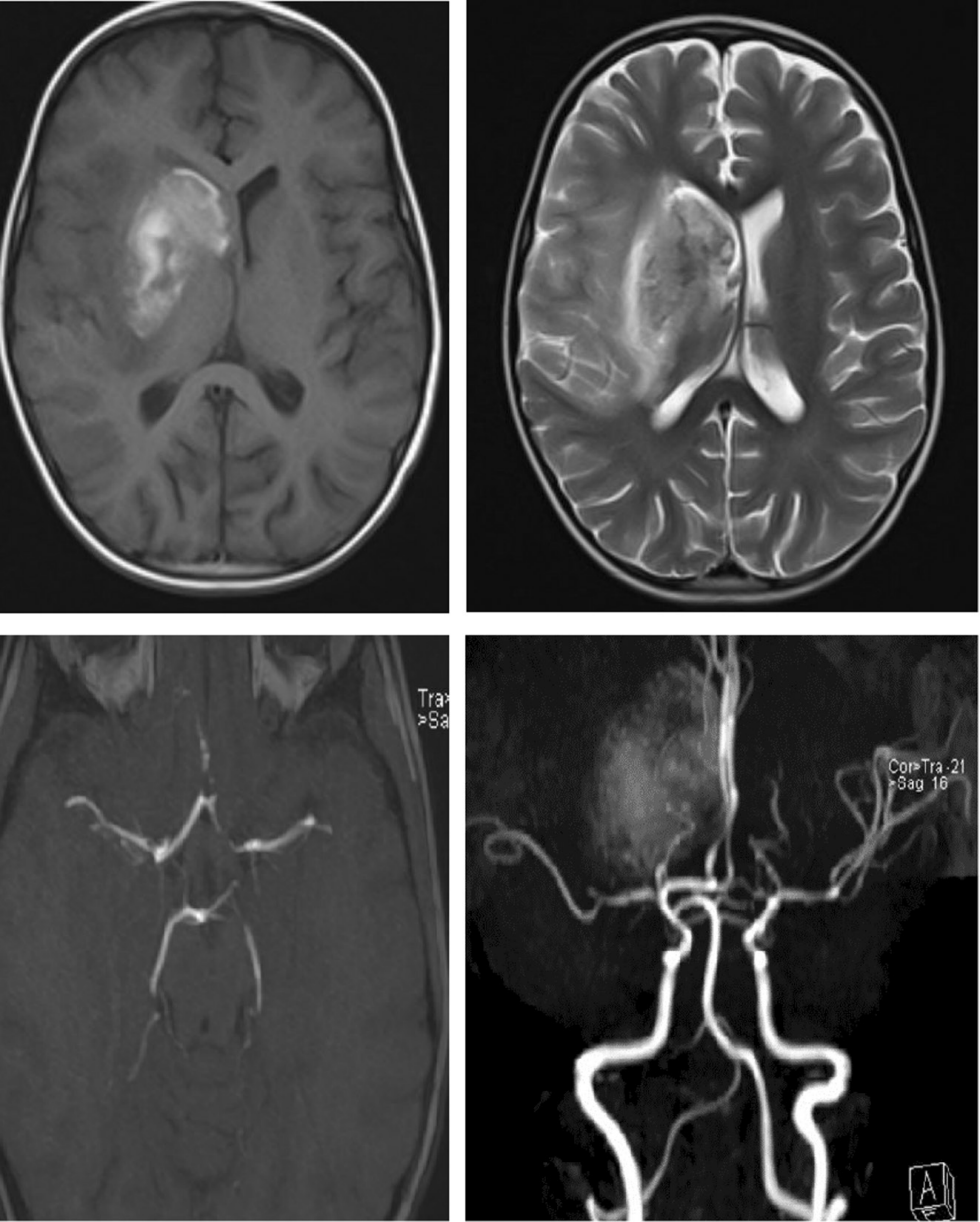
Magnetic resonance angiography (MRA), venography (MRV) and imaging (MRI) findings. Infarction in territory of middle cerebral artery (MCA) is noted with evidence of hemorrhage in right basal ganglia. In MRA filling defect in origin of M1 is noted, also with cutoff sign in the middle and superior branches of MCA at the level of M2. Findings are compatible with thrombosis in MCA and hemorrhagic infarct in the MCA territory.
